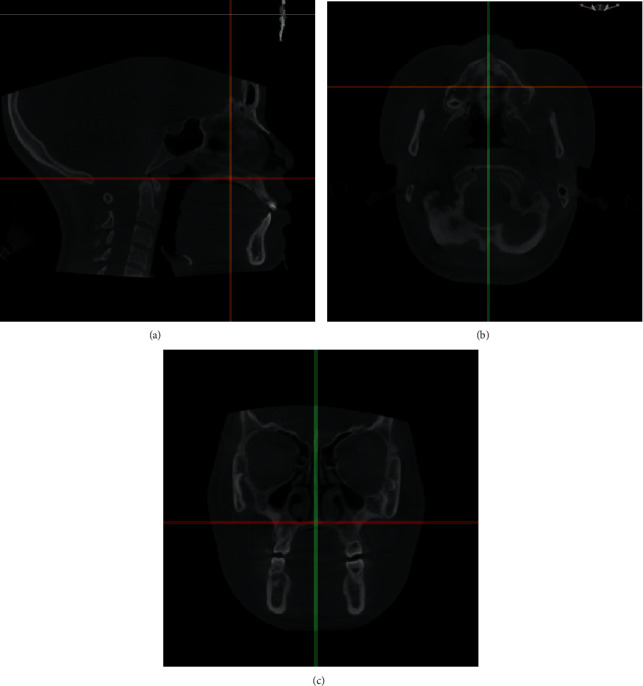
Reorientation of CBCT sections. (a) The sagittal view shows the red line positioned through ANS and PNS which is parallel to the palatal plane, and the yellow line is perpendicular to the red line. (b) Axial view. (c) In the coronal view, the red line is tangential to the nasal floor at its most inferior level.
